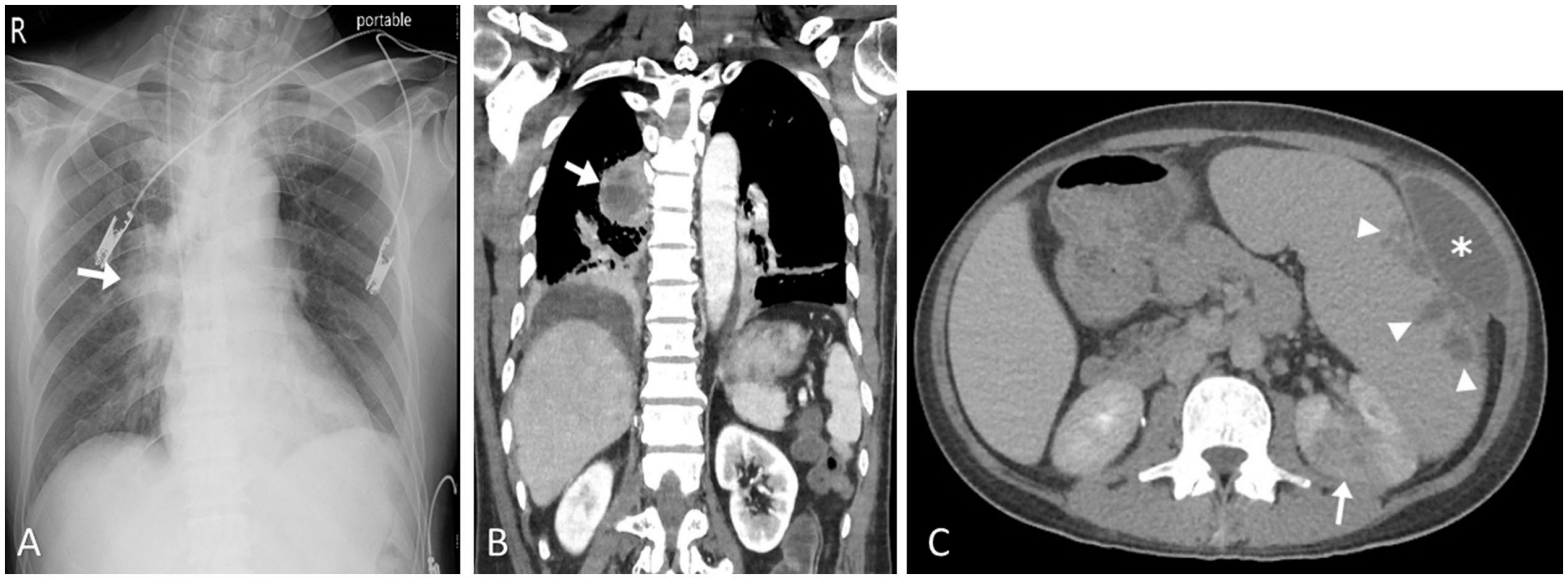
Pulmonary melioidosis of case 1 and splenic abscess and pyelonephritis of case 8. Chest radiograph showed radiopacified lesion in right middLe lung field (arrow) (A). Coronal computed tomography image (soft tissue window setting) confirmed an area of consolidation with cavitation in the right lower lobe, compatible with abscess formation (arrow) (B). Contrast-enhanced axial computed tomography image showed enlargement of the spleen as well as multiple splenic abscesses (arrow heads) and left anterior abdominal wall abscess (asterisk). Left renal cortex also showed focal pyelonephritis (arrow) (C).
